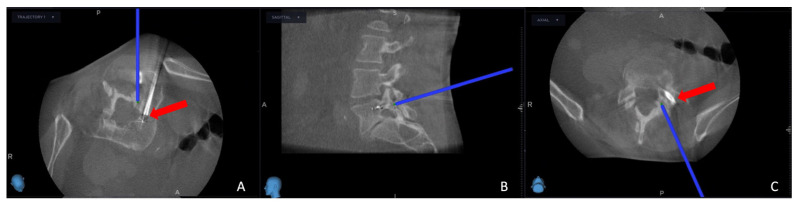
Second intra-operative CBCT scan in the trajectory angled axial view (A), sagittal view (B), and axial view (C). The blue projection line shows the thermostat location, and the red arrow shows the electrode position.
